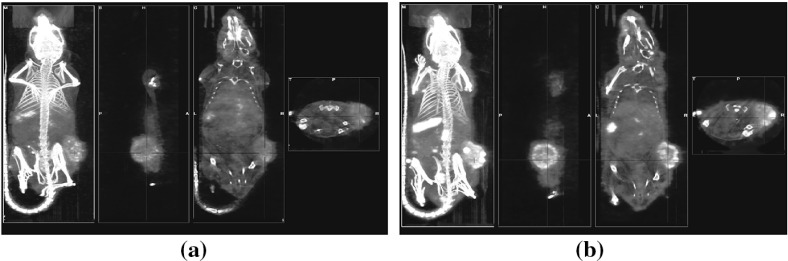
The CT image and sagittal, coronal, and transverse slices of SPECT images for subject T1 imaged at a 24 h and b 72 h. Images are exported from VivoQuant (Release 1.22, Invicro)
